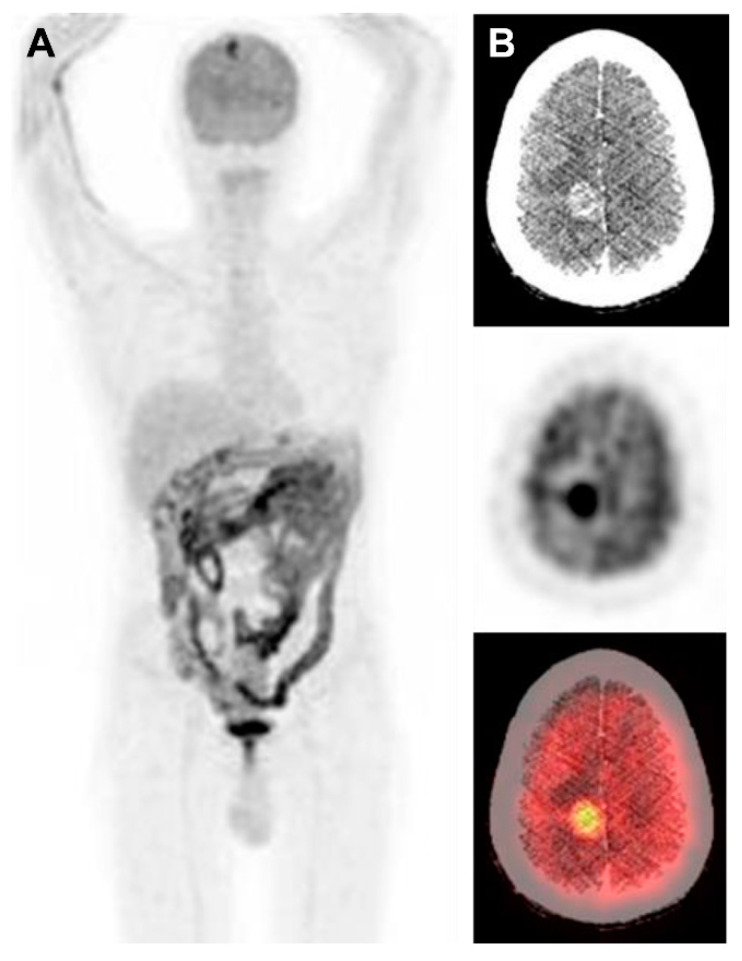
Patient showed no neurologic symptoms nor had been previously treated with steroids, therefore, underwent immunotherapy with ipilimumab in combination with nivolumab [2,3]. During immunotherapy, a reduction of the number and volume of subcutaneous nodules was clinically observed while the patient showed diarrhea, abdominal pain and weight loss likely due to immune-related adverse effects of combined immunotherapy [4]. After the administration of four cycles of immunotherapy with ipilimumab plus nivolumab, a whole-body 18F-FDG-PET/CT scan was performed to evaluate treatment response [5,6]. (A) Maximum intensity projection view; (B) Transaxial CT, PET and fusion images of the brain. The study showed no pathological 18F-FDG uptake in all previously observed sites of disease, with the exception of brain metastases. In fact, the brain lesion in the right parietal lobe, already visible in the pre-immunotherapy 18F-FDG-PET/CT, showed an increase in both size and 18F-FDG uptake, and other smaller brain metastases were detected. Furthermore, intense and diffuse 18F-FDG uptake was visible in the bowel likely due to enterocolitis induced by immunotherapy [5,7]. Based on 18F-FDG-PET/CT results, MRI of the brain was prescribed [8].
